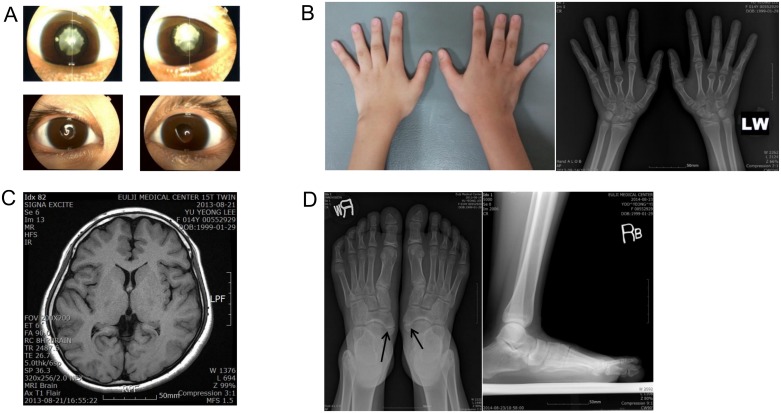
Clinical findings of the proband and preoperative eyes of the father. (A) In the upper panel, both of the father’s eyes showed white congenital cataracts before preoperative slit-lamp images were obtained, and the lower panel shows slit-lamp images for both of the proband’s eyes, showing postoperative bilateral phacoemulsification with posterior chamber lens implantations. (B) Brachydactyly on both hands was observed and hand X-rays revealed short 3rd and 4th left metacarpals and a short right 3rd metacarpal. (C) Macrocephaly without definite brain anomalies on 1.5-tesla magnetic resonance imaging. (D) Foot radiographs showing flat feet and both accessory navicular bones.
